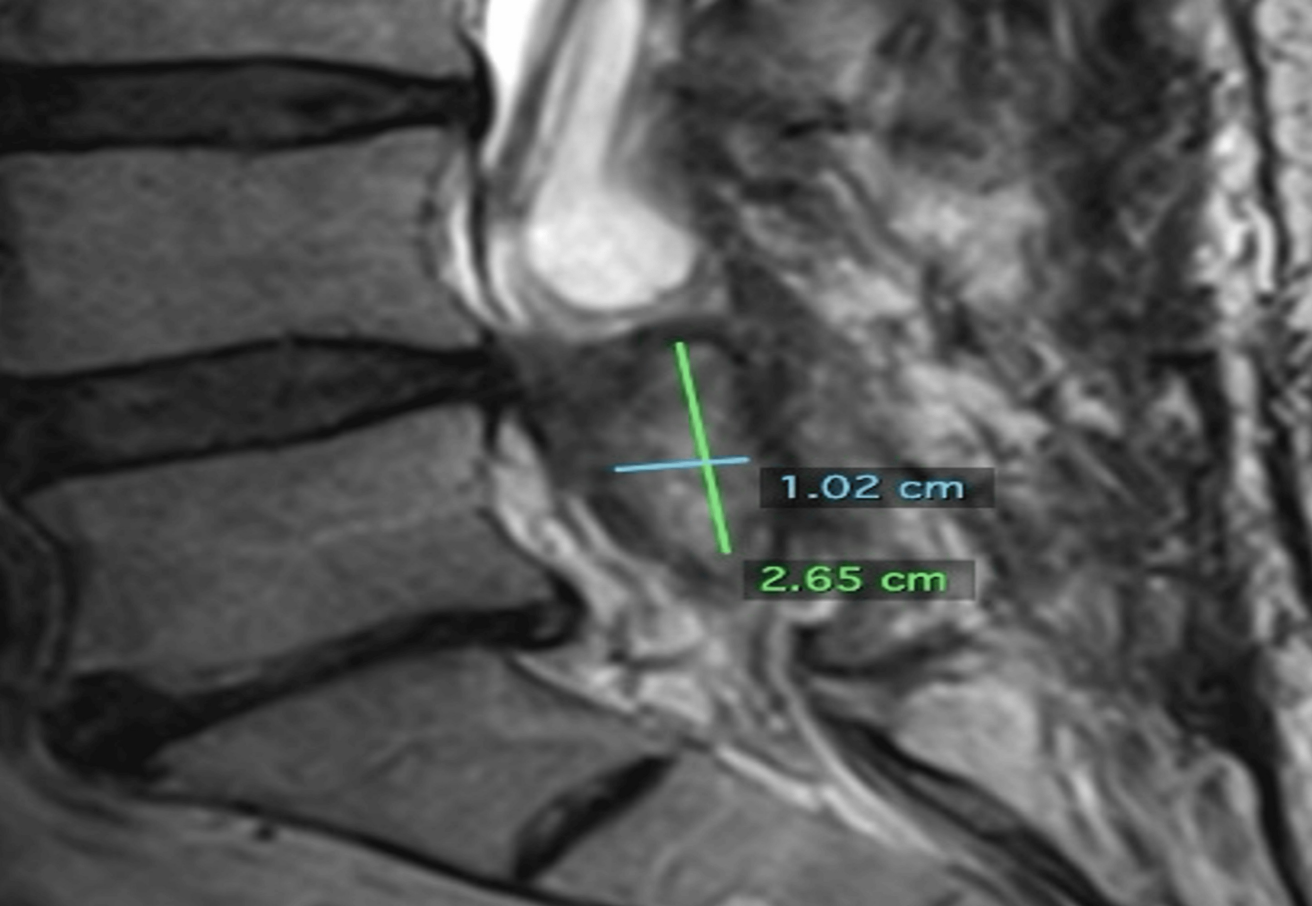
Sagittal image from an MRI L-spine with contrast dated November 7, 2023, approximately five months post cryoablation. The tumor to measure 2.65 cm superiorly to inferiorly and 1.02 cm anteriorly to posteriorly.
